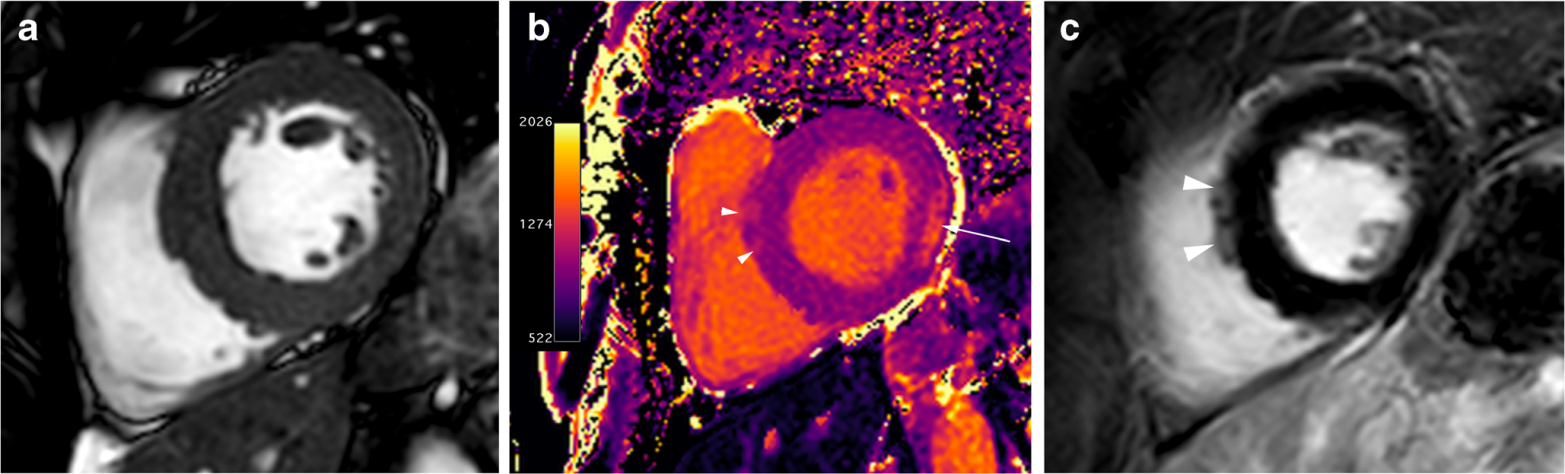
T1 mapping in a 60-year-old man with septal HCM. a, End-diastolic short-axis SSFP cine MR image showing septal myocardial hypertrophy (20 mm). b, Short-axis non-enhanced MOLLI (modified look locker inversion recovery) (1,5 T) T1 map demonstrates patchy areas of prolonged T1 values in the anteroseptal segment (arrowheads; 1203 ms) and in the lateral wall (arrow; 1343 ms). At 1.5 T, normal myocardium has a T1 relaxation time of 940–1000 ms. c, Short-axis LGE MR image through the same plane shows small patchy myocardial enhancement at anteroseptal segment (arrowheads)
